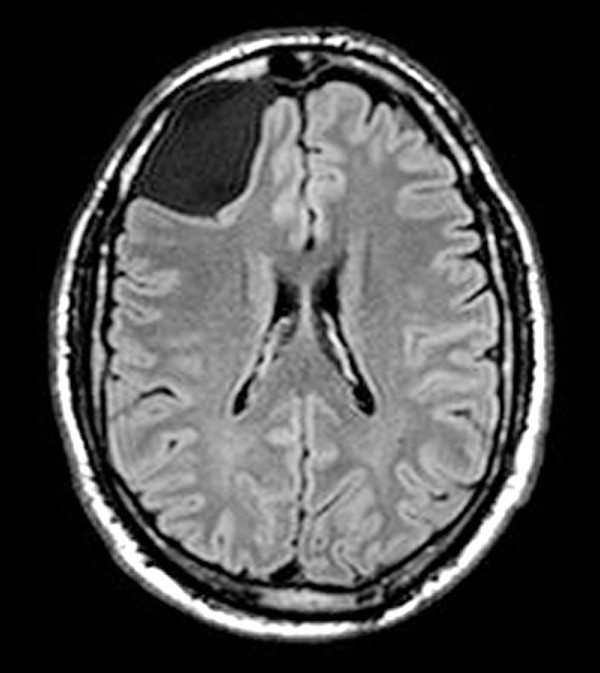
T1-weighted MRI image. A large arachnoid cyst deforms right frontal lobe; outer membrane of cyst is faintly visible. This patient presented with headache, vertigo, and seizures. The cyst wall consisted of respiratory-like epithelium.
